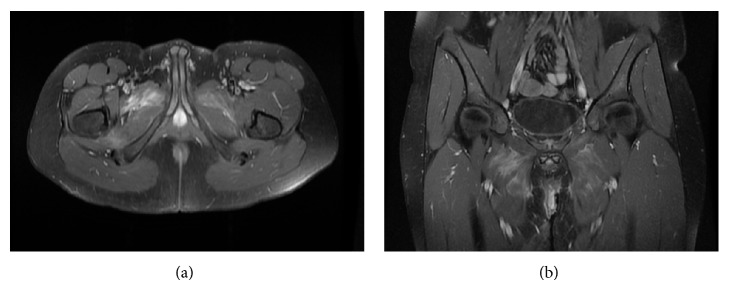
Postoperative MRI (a) axial, (b) coronal showing no collection and just residual edema and myositis 6 months after drainage.
